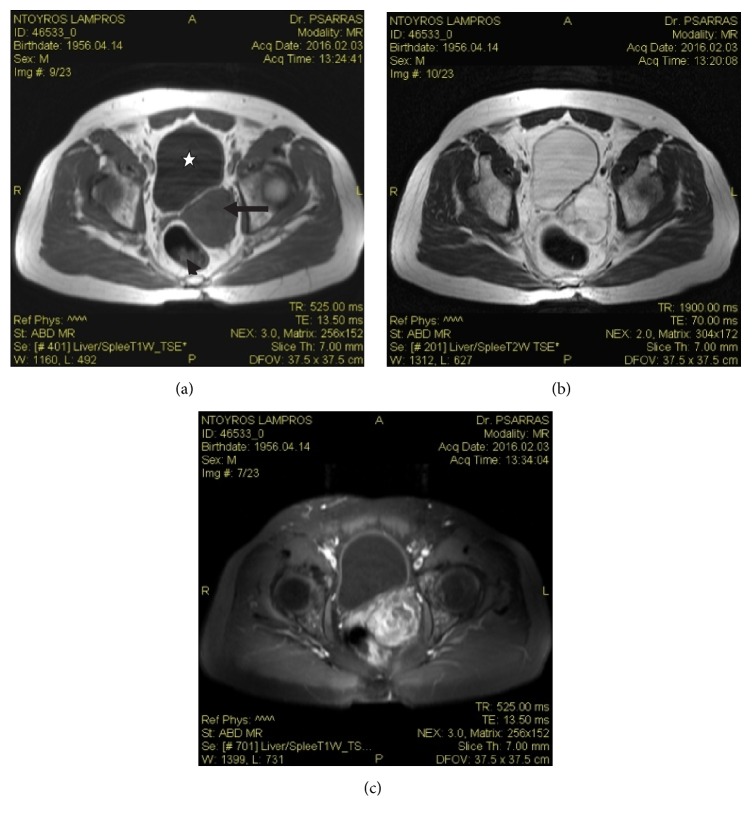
(a) T1-weighted axial MRI image demonstrating a fusiform, lobed, well-circumscribed, hypointense lesion (arrow) with regular borders, compressing the rectum (arrowhead) and the posterior wall of the urinary bladder (white star). (b) T2-weighted axial MRI image showing the lesion having hyperintense signal and containing thin septa. (c) T1-weighted postcontrast axial MRI demonstrating heterogeneous enhancement of the lesion's lobules after intravenous administration of paramagnetic contrast agent.
